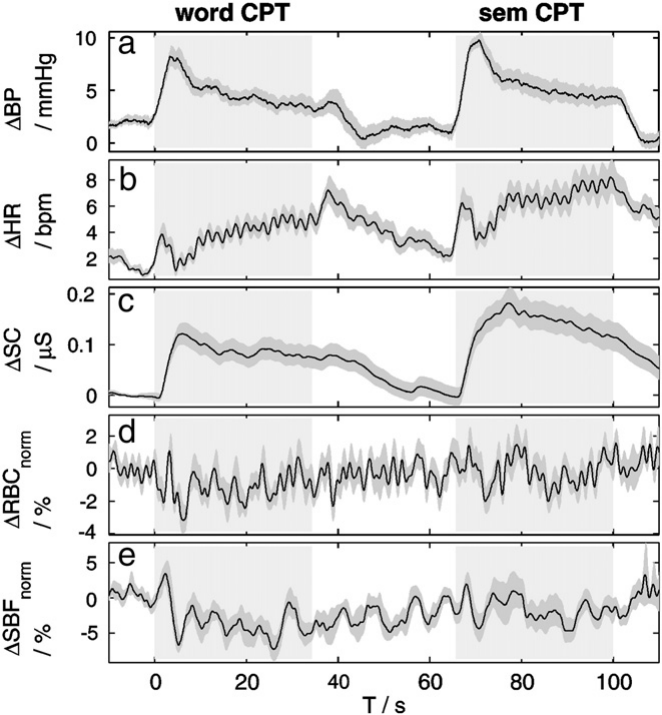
Task-evoked changes in (a) blood pressure, (b) heart rate, (c) skin conductance, (d) red blood cell concentration changes and (e) scalp blood flow measured by Laser Doppler. The time traces are averaged over all subjects and all block repetitions. The periods of the word-CPT and sem-CPT tasks are indicated with grey blocks. The grey bands indicate the standard error of mean.
